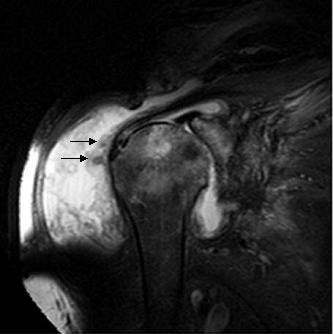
Coronal fat-suppressed T2-weighted magnetic resonance image of the left shoulder revealing diffuse synovitis and bursitis with a large joint effusion. Numerous loose bodies representing rice bodies and degenerative cystic changes within the humeral head were also present (arrow).
